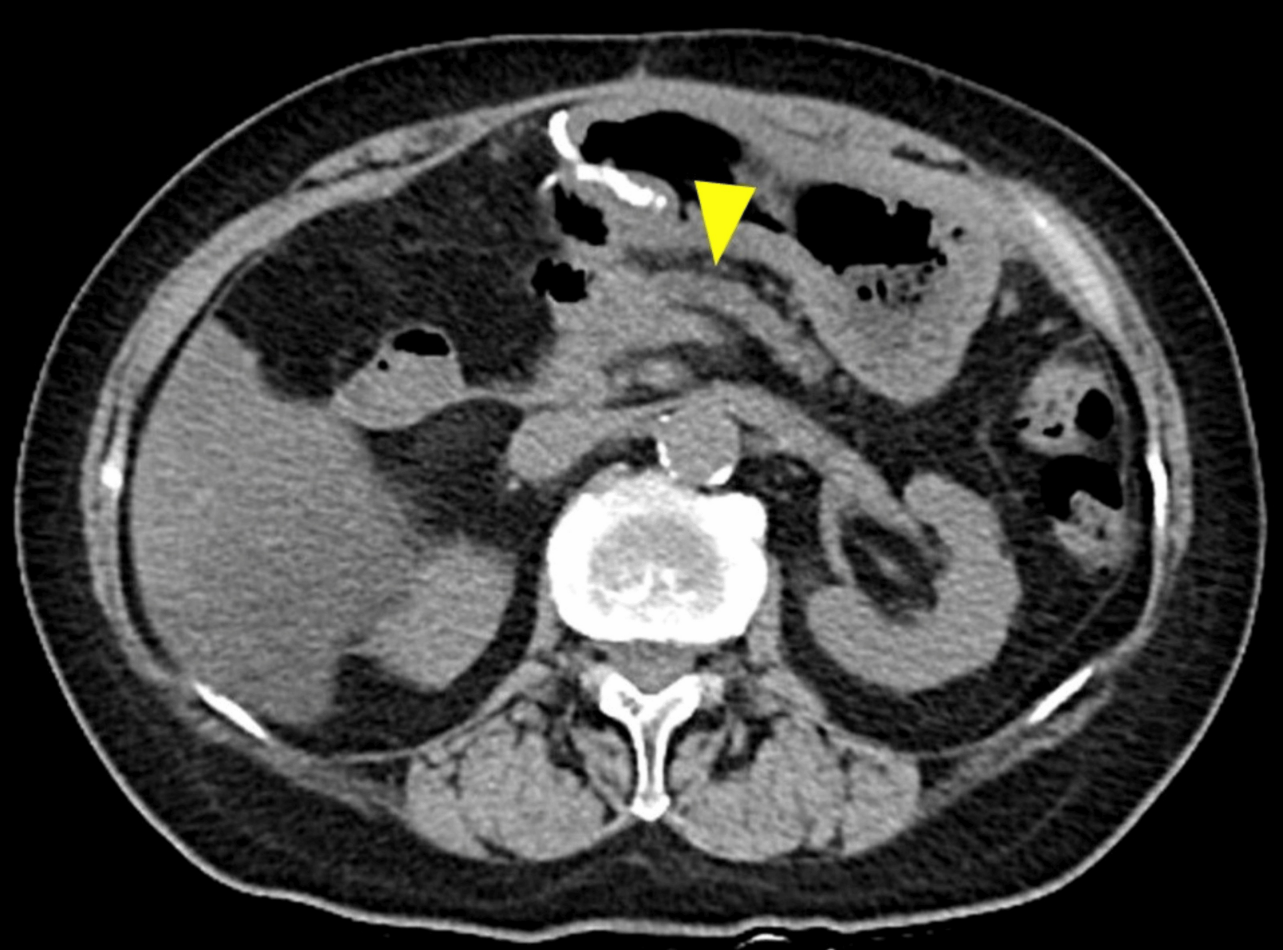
Plain abdominal computed tomography of Case 1 performed four months after the initial surgery. Computed tomography showed no pancreatic stent and no evidence of intra-abdominal fluid collection (arrrowhead: pancreas).
